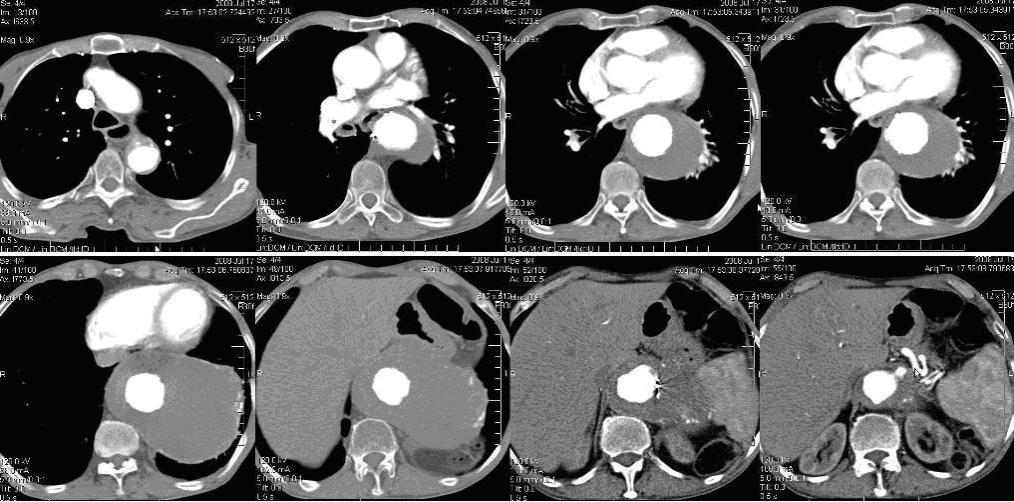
Computed tomography scan showing the patient's Crawford Type I thoracoabdominal aneurysm.
